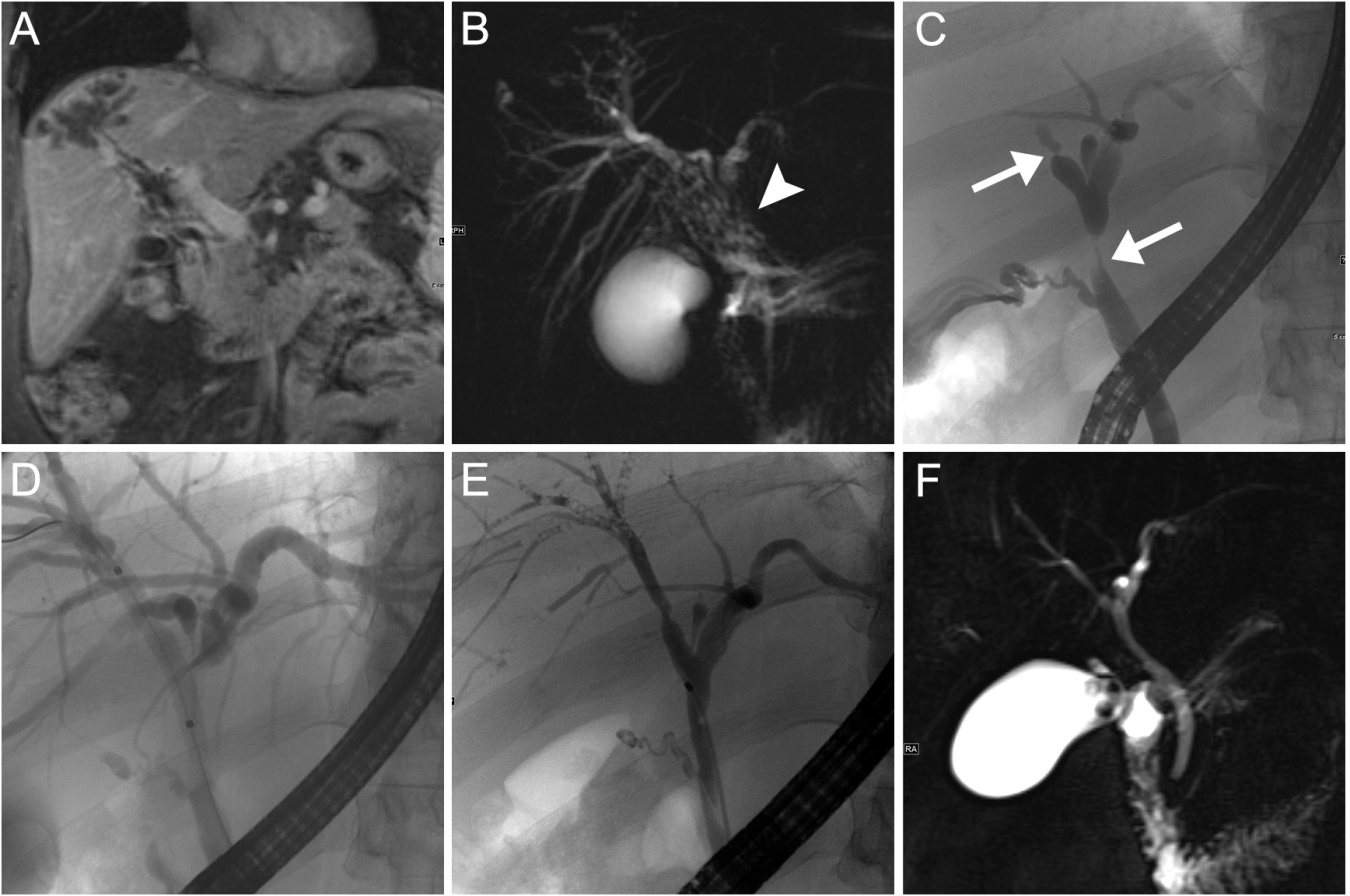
Imaging and ERC findings patient 2. Contrast-enhanced MRI in coronal orientation shows a peripheral liver mass with contiguous perivascular infiltration towards the liver hilum (A). MRCP shows central bilateral bile duct stenosis due to a mass lesion of the liver hilum with micro vesicular appearance (B, arrow head). ERC before (C) und during (D) balloon dilation confirms central stenosis of main and right hepatic ducts (arrows). After serial ERC with balloon dilatation satisfactory remodeling of bile ducts is visible at ERC (E) and MRCP (F).
